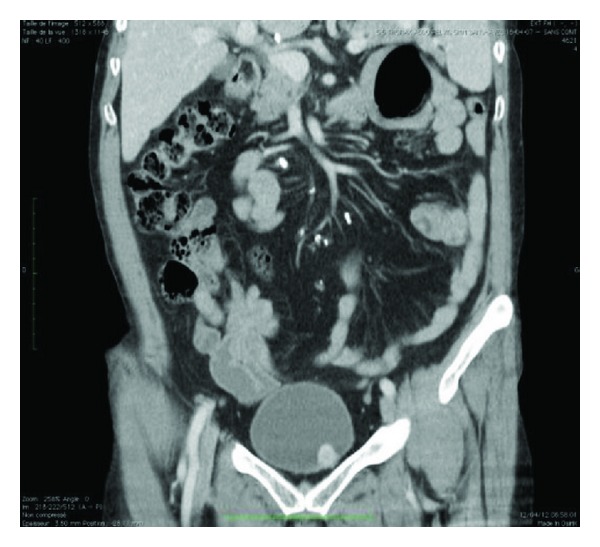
CT scan showing small tumour located in the anterior front of the bladder with well-defined bounds and increasing its density after the injection of iodine-containing contrast solution.
